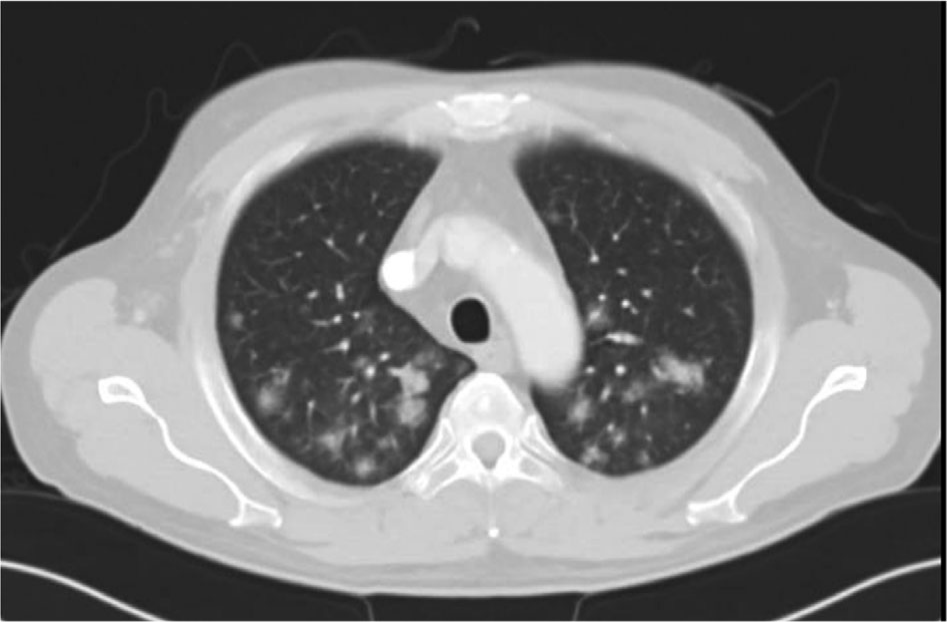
Computed tomography scan of the thorax revealing multiple halo sign shadows in the lung field
